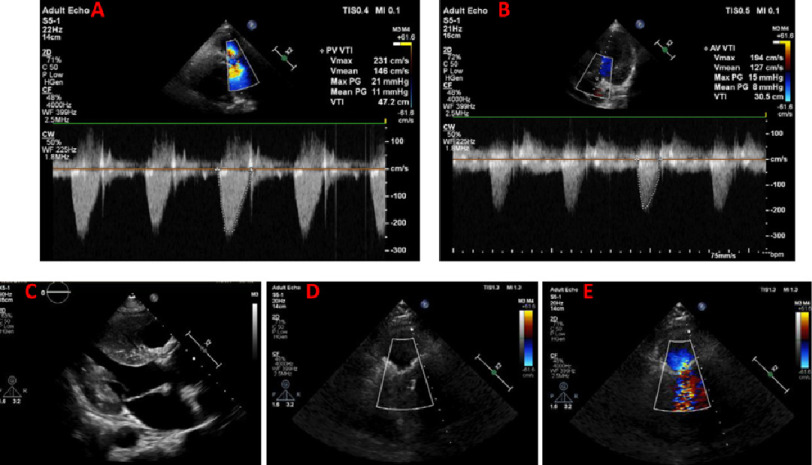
Continuous wave Doppler over neo-pulmonary valve showing no significant gradients across valve 1-month post-surgery (A). Continuous wave Doppler over neo-aortic valve showing no significant gradients across valve 1-month post-surgery (B). 2D echocardiogram of the neo-aortic valve (Freestyle prosthesis) with full leaflet motion (C). RV outflow view showing the neo-pulmonary valve with full leaflet motion in 2D (D) and color Doppler showing laminar flow across the valve (E).
